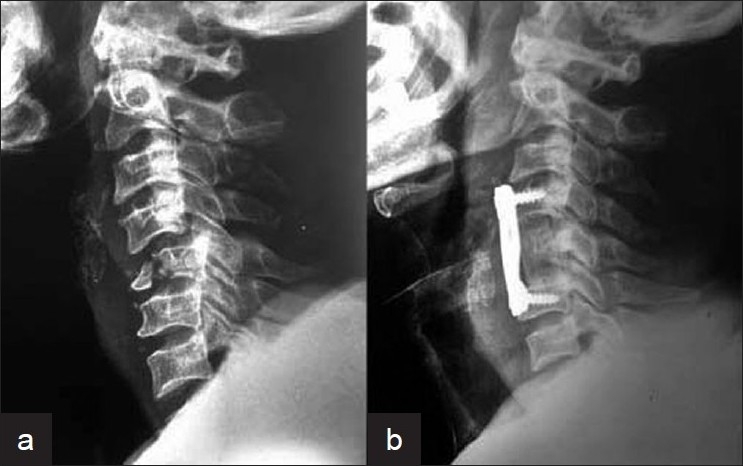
X-ray cervical spine (lateral view) showing (a) C5 fracture with subluxation. (b) C5 fracture with subluxation treated with anterior corpectomy, fusion with fixation (21 months post-operative)
